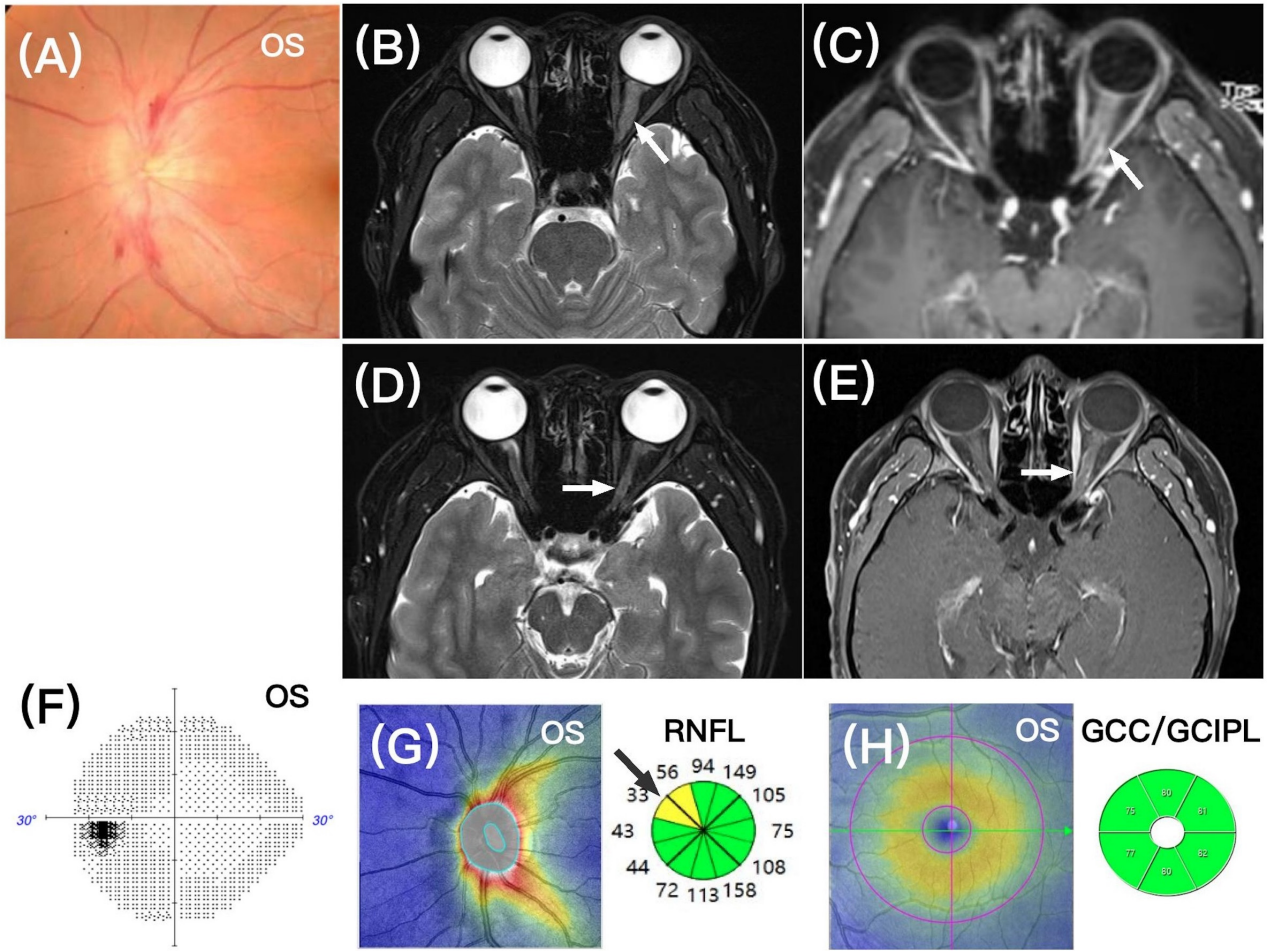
(A) Fundus photograph showing mild optic disc elevation in the left eye with blurred borders and surrounding hemorrhages. (B,C) Orbital MRI showing thickening of the left optic nerve in the orbital segment, with enhancement of the nerve sheath, respectively (white arrows). (D,E) One month after treatment, orbital MRI showed improvement in optic nerve thickening and reduced enhancement, respectively (white arrows). (F) One year follow-up visual field (HVF) test showed normal findings in the left eye. (G,H) One-year follow-up optical coherence tomography (OCT) showed very mild focal thinning of the peripapillary retinal nerve fiber layer (RNFL) in the left eye (black arrow, sectors in yellow color), while the ganglion cell complex/ganglion cell-inner plexiform layer (GCC/GCIPL) appeared normal.
